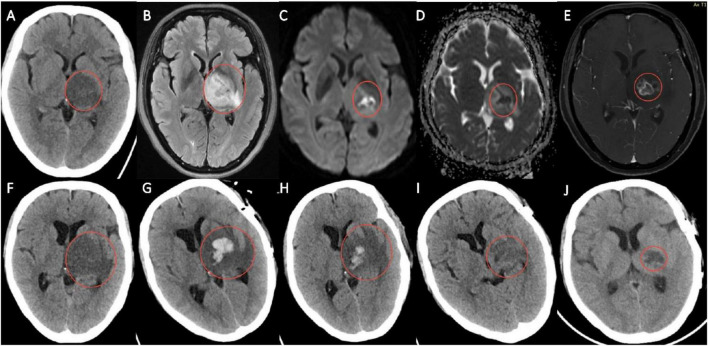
(A) Head CT at admission. (B) Head MRI scan. (C,D) Head Diffusion-weighted imaging (DWI) scan. (E) Contrast-enhanced MRI. (F) Head CT scan at the point of disease progression. (G) Post-operative head CT scan. (H–J) Follow-up head CT.
